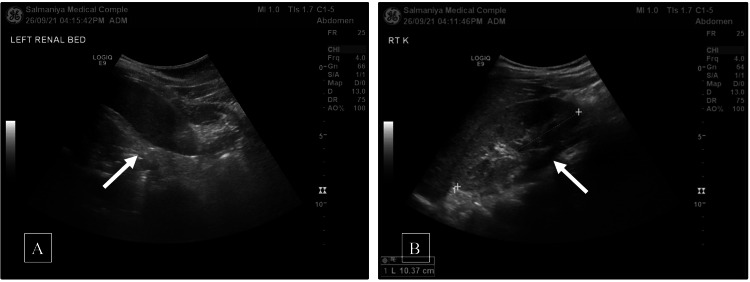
(A) Abdominal ultrasound of an 11-year-old female patient with 17q12 deletion syndrome showed empty left renal fossa (see arrow), and (B) normal right kidney in the same patient (see arrow).
